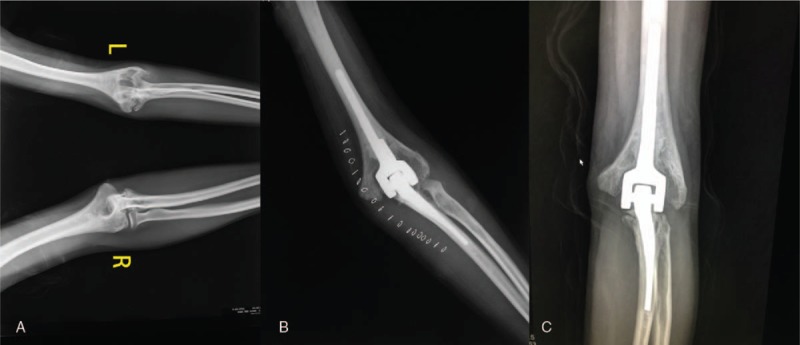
(A) Preoperative anterior radiograph of bilateral elbow joints, revealing end-stage arthropathy with narrowing of the joint space, erosions of the articular facets, and bone deformation of left elbow compared with the right elbow. (B) Anterior radiograph of the left elbow joint 3 months after left total elbow arthroplasty. (C) Anterior radiograph of the left elbow joint 5 years after the operation.
